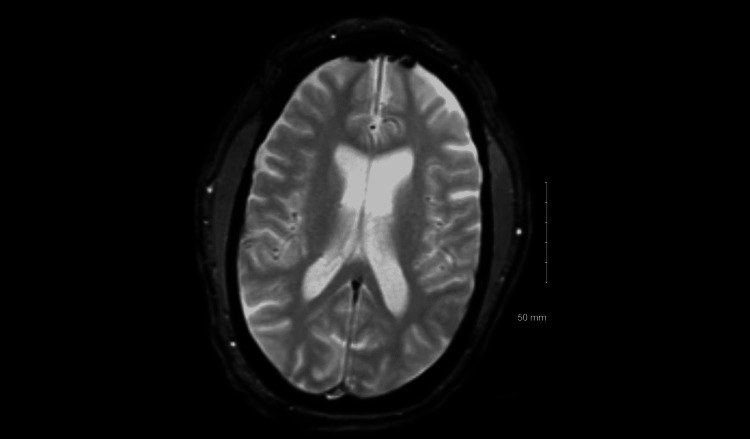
MRI of the head. Magnetic resonance imaging (MRI) did not show any evidence of edema, hemorrhage, infarct, hydrocephalus, or neoplasm.
